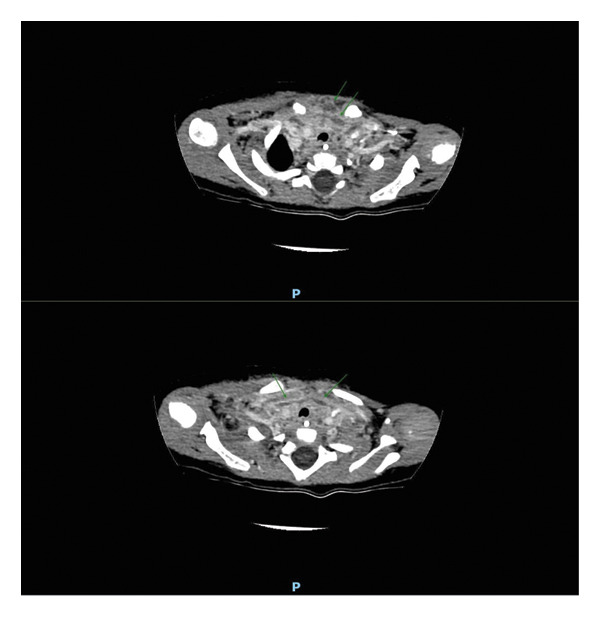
CT image from Case 2 obtained on day 9, showing multiple poorly defined mediastinal fluid collections indicated by the green arrows. P, posterior.
